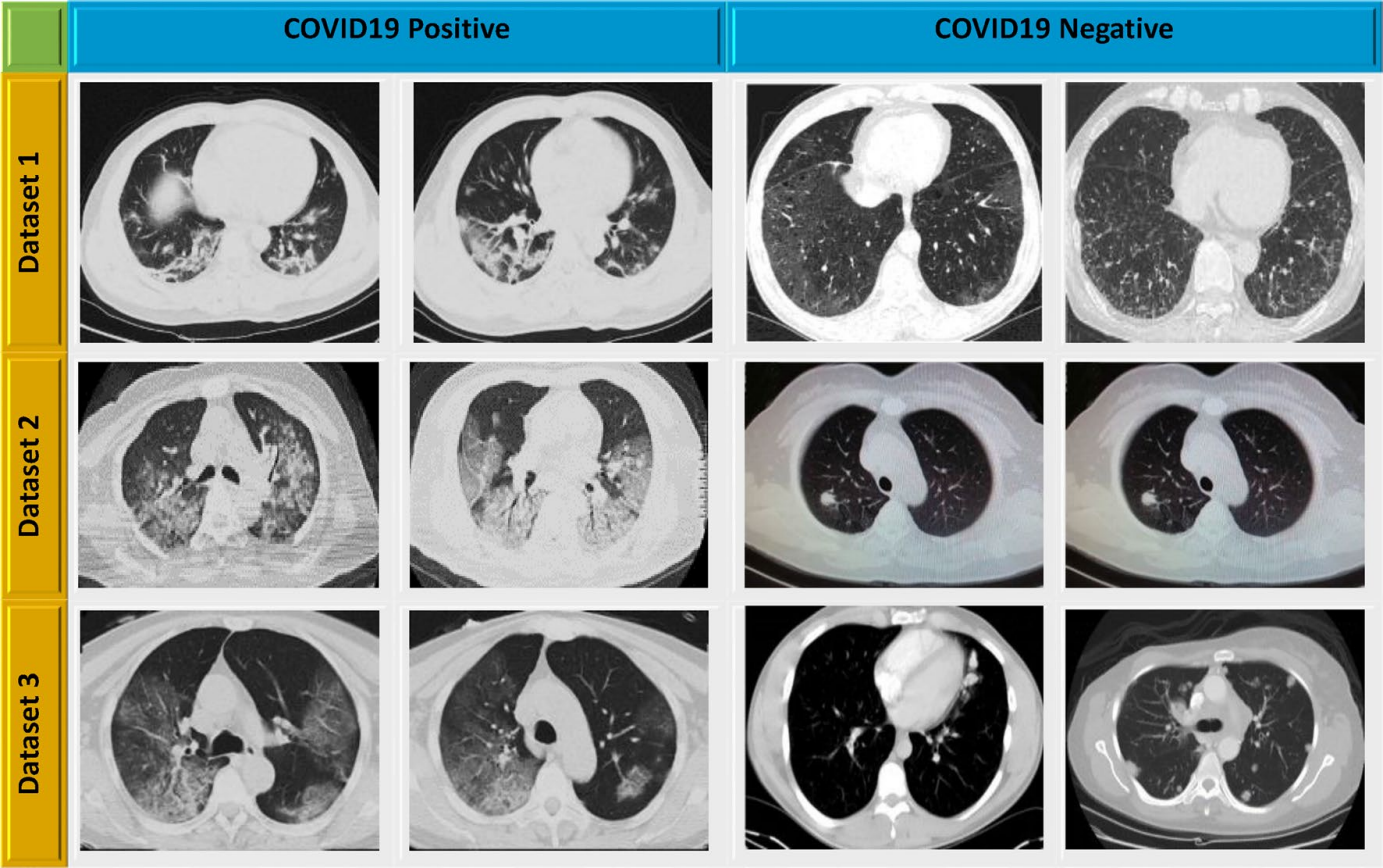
Sample CT scan images from three datasets.
